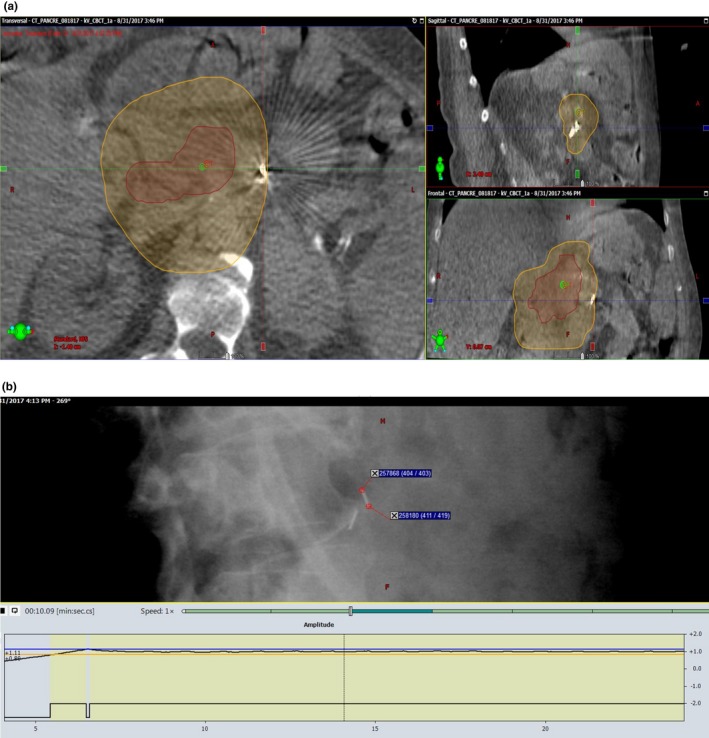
A fiducial marker in the setup CBCT image (a) and its corresponding location in the intrafraction IMR image (b). The contours shown in (a) corresponded to two prescribed dose levels, 45 Gy (orange) and 75 Gy (red), respectively. The pixel information was recorded for both tips of the fiducial in each IMR image.
